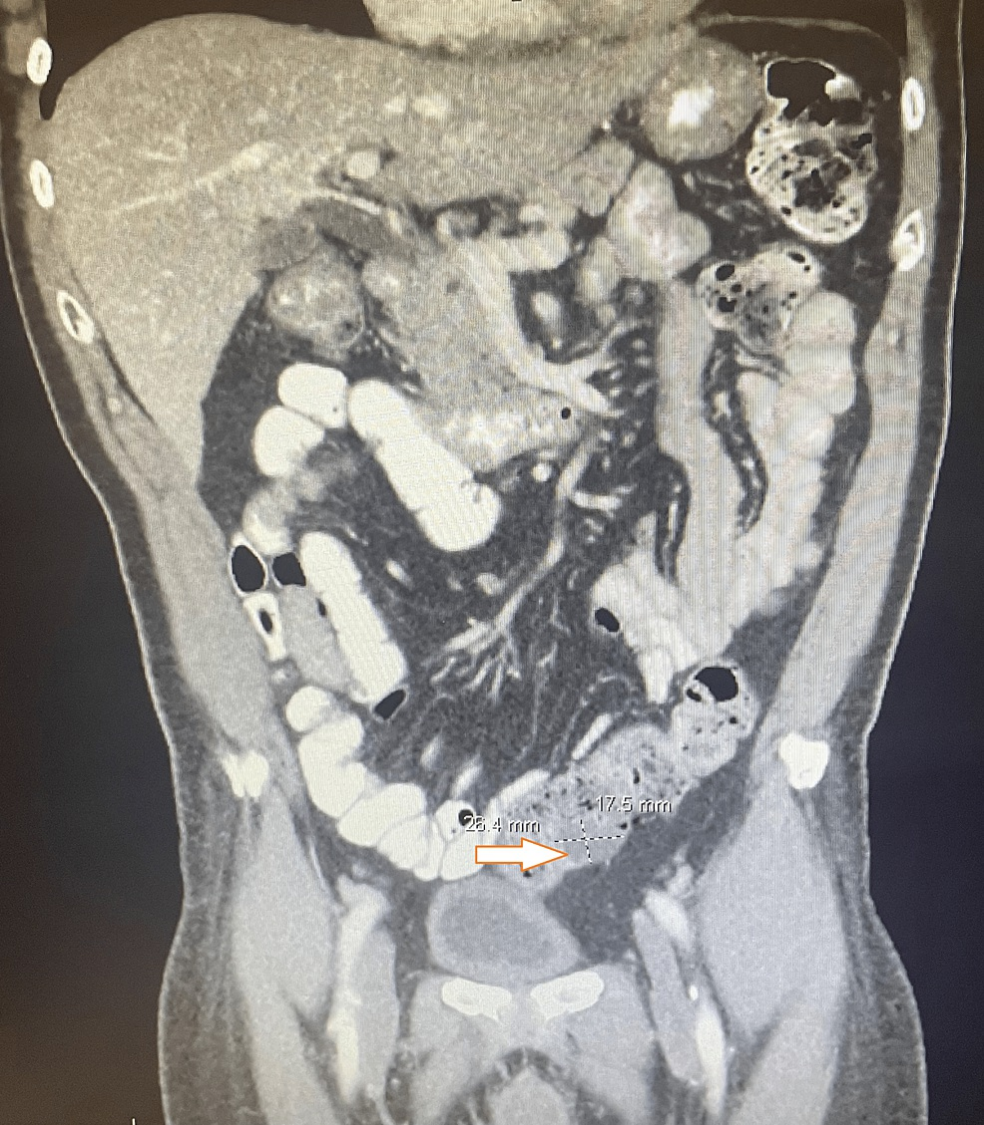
CT scan of the abdomen and pelvis Triangular soft tissue opacity in the sigmoid colon measuring about 26 x 18 mm on coronal image CT: computed tomography
